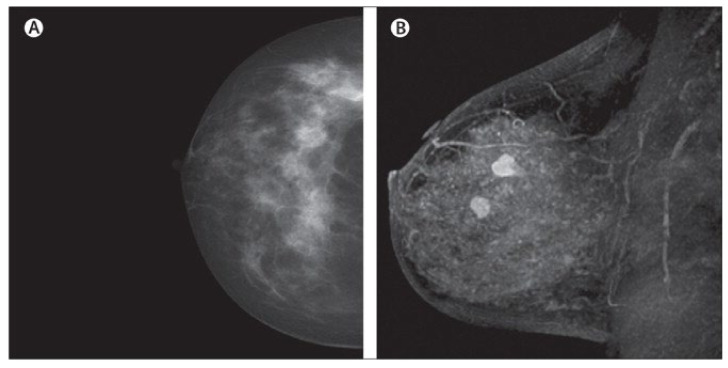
Dense cancerous breast tissue images conducted by MRI method from different angles. (A) Normal; (B) malignant [82]. Reprinted/adapted with permission from [82]. 2011, Elsevier.
